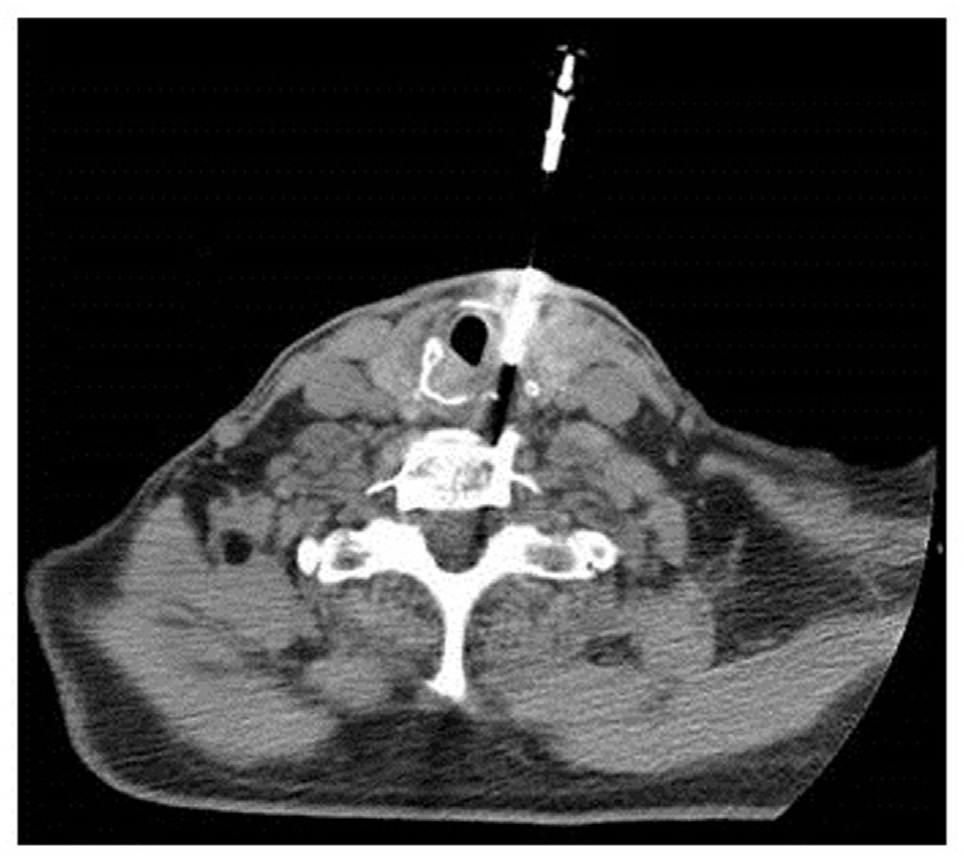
CT-guided biopsy of the mass in the cricoid cartilage.
